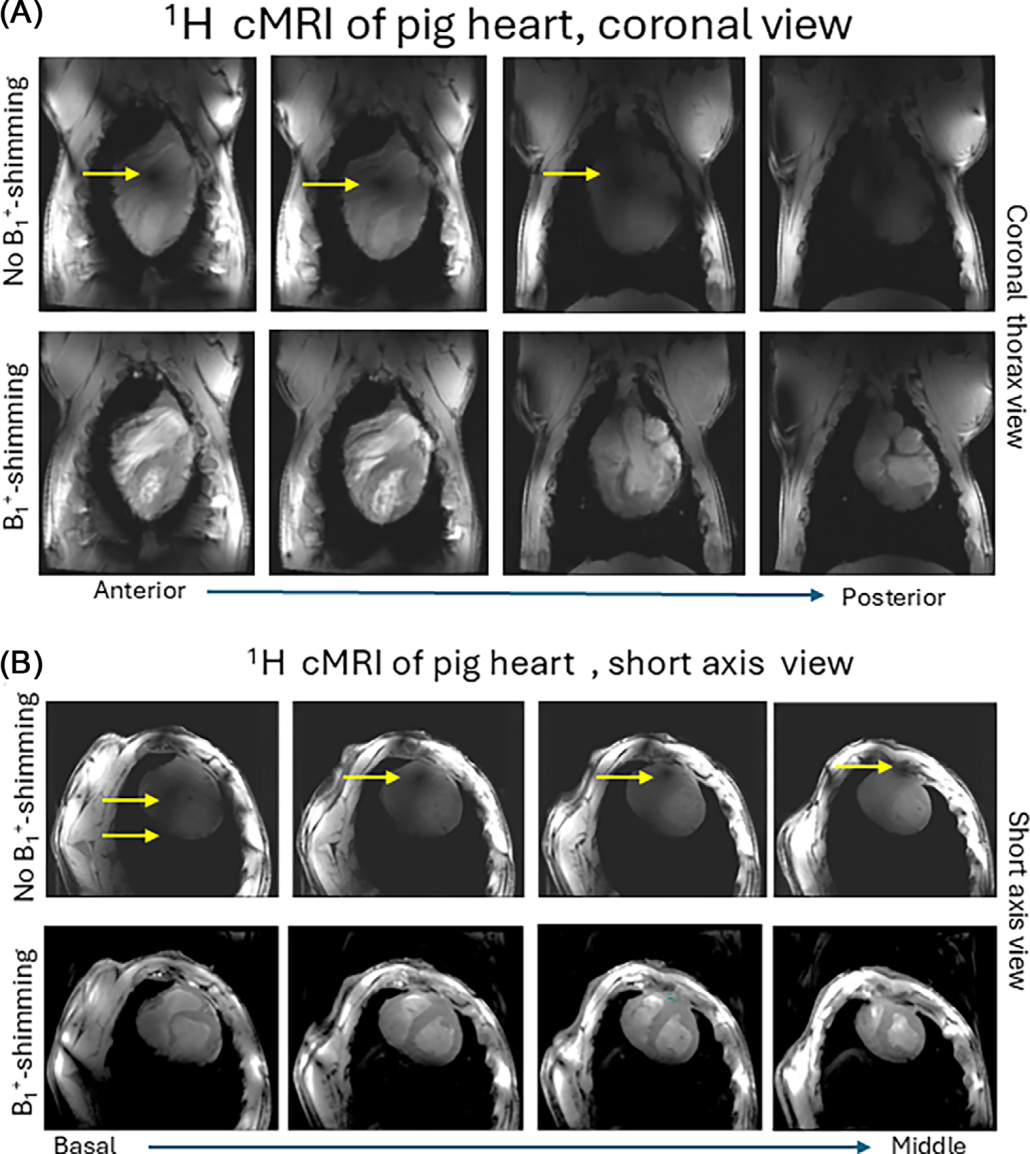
Cardiac MRI using ^1^H‐array. The efficiency of the subject‐specific B_1_+‐shimming is confirmed by the elimination of the destructive interferences and a significant increase of Tx‐efficiency on both coronal planes (A) and short‐axis view images (B). The suppression of B_1_
